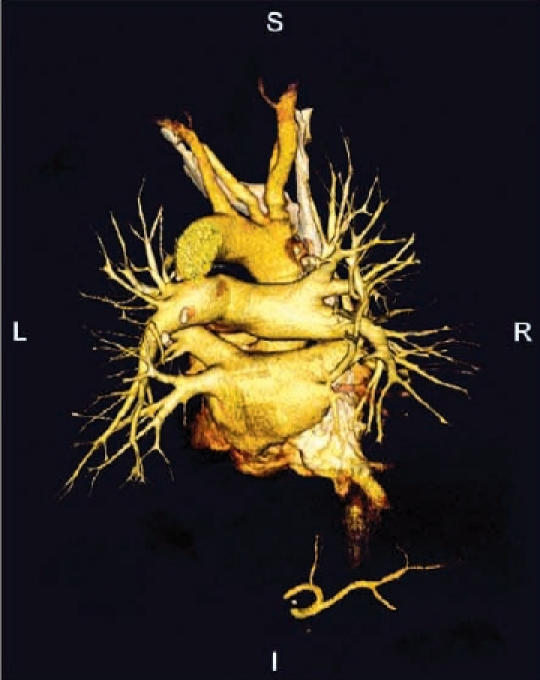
Multislice CT demonstrating aortic (above), pulmonary arterial (mid) and pulmonary venous vasculature (posterioanterior projection)
